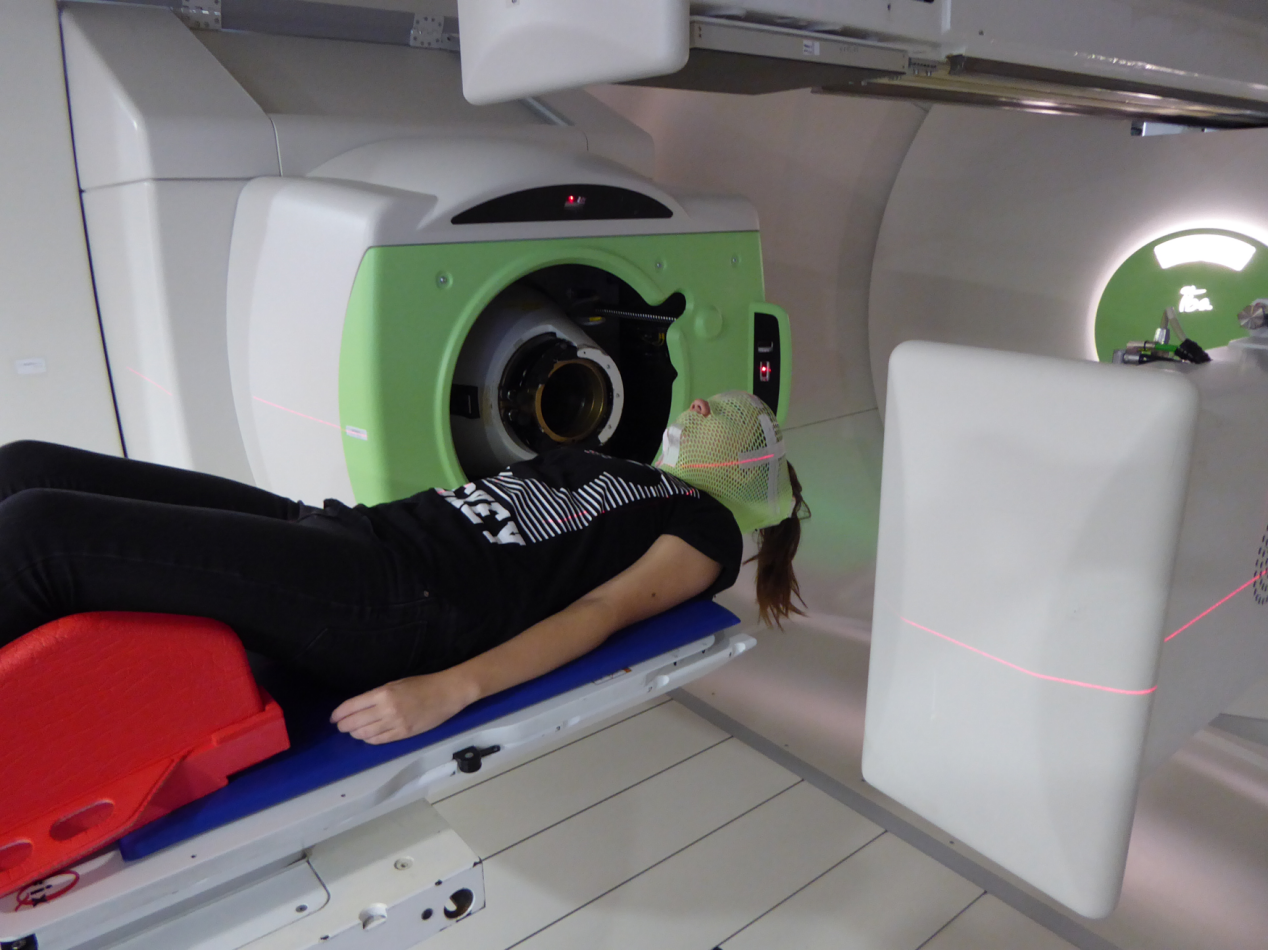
Photo of the inner part of the gantry with proton nozzle (left), treatment couch, extended flat x-ray panels, and a patient with head immobilizing mask (light green color). The red LASER light for initial patient positioning is turned on.
